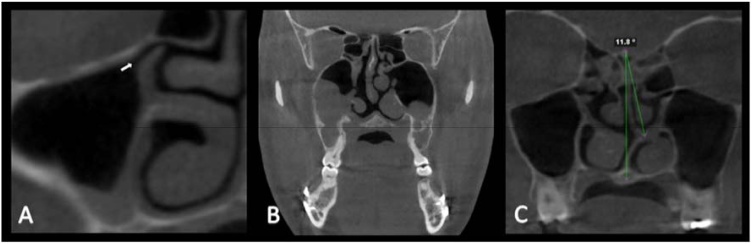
(A) CT image (coronal section) showing position of right maxillary ostium. (arrow). (B) CT image (coronal section) showing thickening of maxillary sinus mucosa bilaterally. (C) CT image (coronal section) showing nasal septum deviation using angular measurement tool of Dolphin Imaging software.
